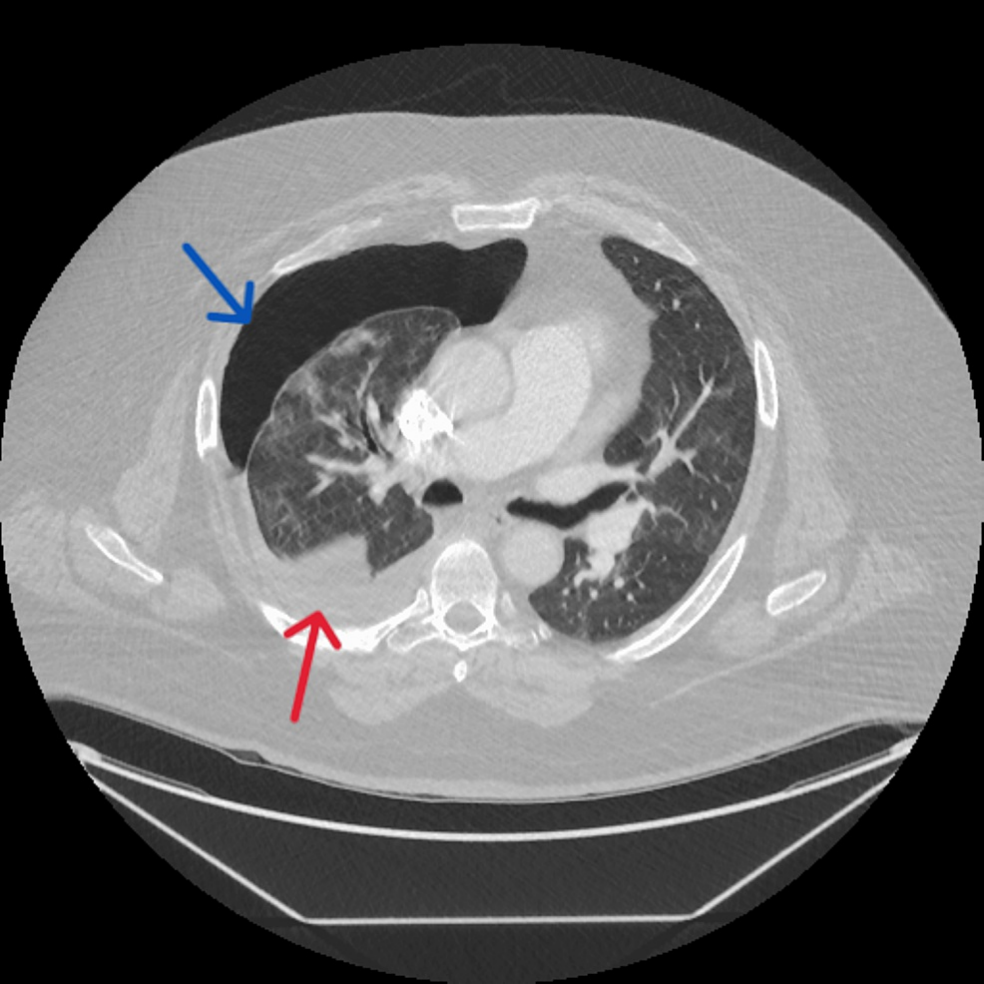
Computed tomography angiogram (CTA) chest The blue arrow shows that 40-50% right-sided pneumothorax has developed without obvious leftward cardio mediastinal shift. The red arrow shows moderate-sized layering right pleural effusion.
